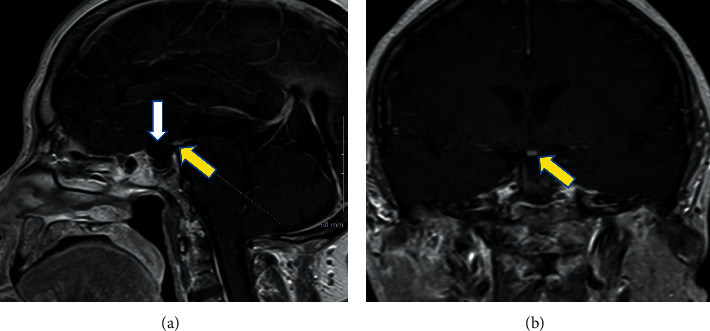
MRI brain with contrast showing empty sella with complete absence of anterior and posterior pituitary glands/infundibulum and tissue representing an ectopic hypothalamic-pituitary gland. (a) Sagittal T1 section. (b) Coronal T1 section (white arrow shows the empty sella, and yellow arrows depict the ectopic pituitary gland).
